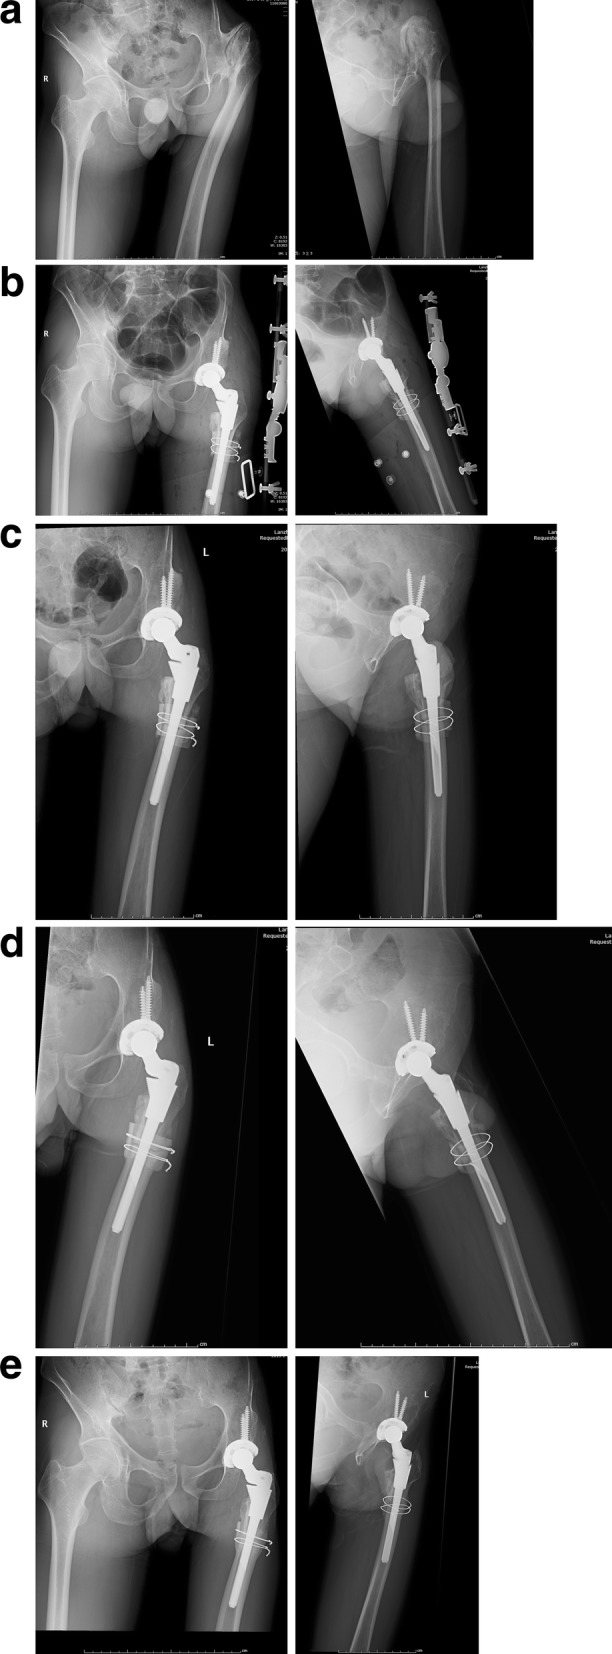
Example case of a 42-year-old male patient. This patient had left Crowe type IV DDH and a Harris hip score of 42 points. a1–a2 Preoperative pelvic radiograph showing significant superior dislocation of the left femoral head forming a joint with a false acetabulum, left DDH, and subtrochanteric PFD. b1–b2 Immediate postoperative AP pelvic and lateral femur radiographs after total hip arthroplasty with osteotomy and modular S-ROM prosthesis, resulting in acetabular cup implantation at the true acetabulum level, with excellent intramedullary filling of the prosthesis, and fixation effect of intramedullary nail at the proximal and distal osteotomies by the distal and proximal segments of the prosthesis. c1-c2 At 3 months postoperatively, the AP pelvic and lateral femur radiographs showed that the femoral osteotomy was in the stage of osteophyte formation and the prosthesis stem was well filled. d1–d2 At 9 months postoperatively, the AP pelvic and lateral femur radiographs showed that the acetabular and femoral prosthesis were stable, and the femoral osteotomy continued to heal. e1–e2 AP hip radiographs at 3 years postoperatively showed good position of the prosthesis (without prosthesis subsidence), excellent healing of the osteotomy surfaces, and no limb-length discrepancy. d Lateral radiograph taken at 1 year postoperatively. Note: DDH, developmental dysplasia of the hip; PFD, proximal femoral deformity; AP, anteroposterior
